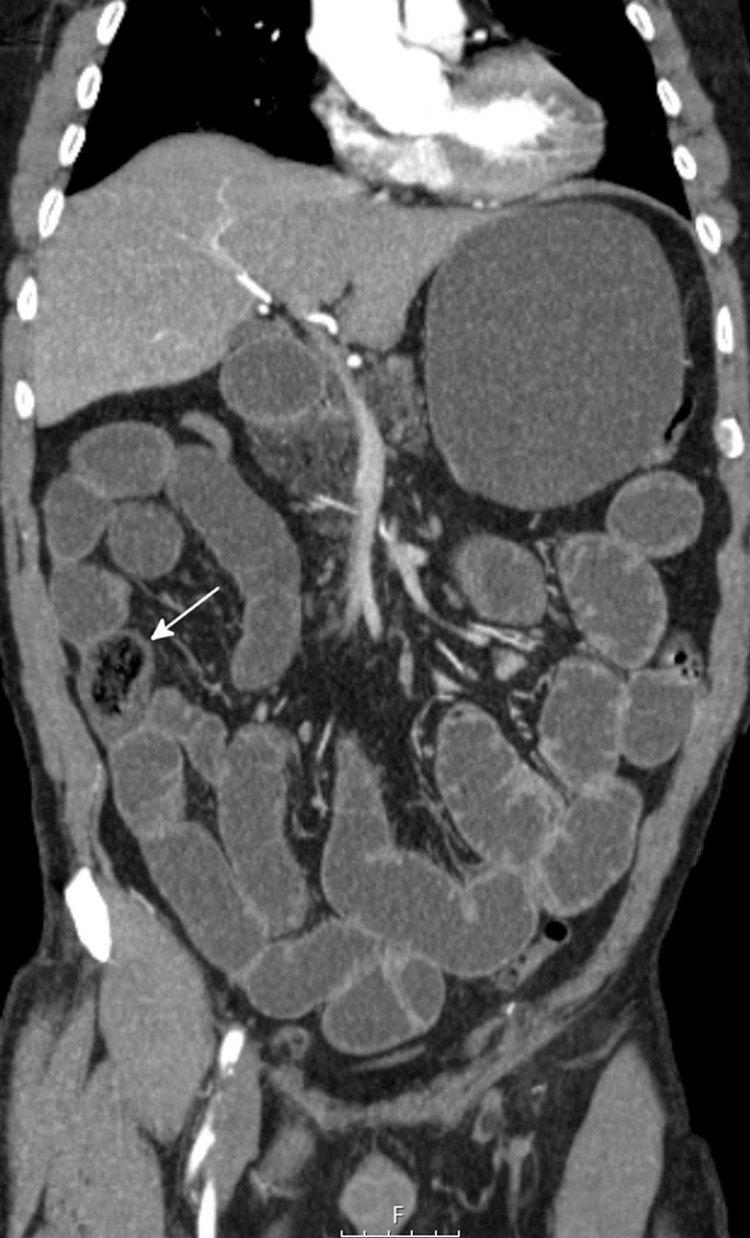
Abdominopelvic computed tomography with angiography scan of the abdomen and pelvis showing an endoluminal nodular image in the distal ileum compatible with a bezoar (arrow).
